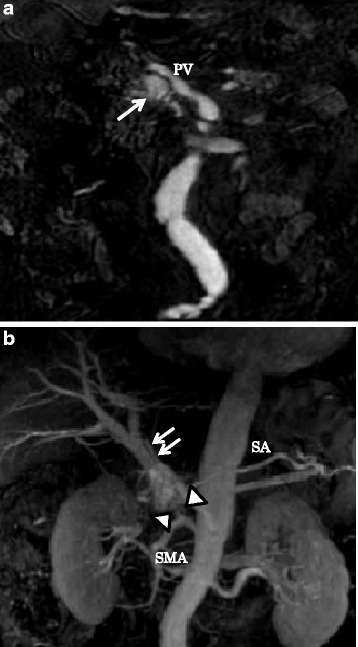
Magnetic resonance imaging showed strong enhancement of the conglomeration in the pancreatic head (*single arrow*; a). Reformed maximum intensity projection imaging showed feeders from the superior mesenteric artery (SMA) (*arrow heads*) with the early appearance of the portal vein (*double arrows*) in the arterial phase (b). *PV* portal vein, *SA* splenic artery
